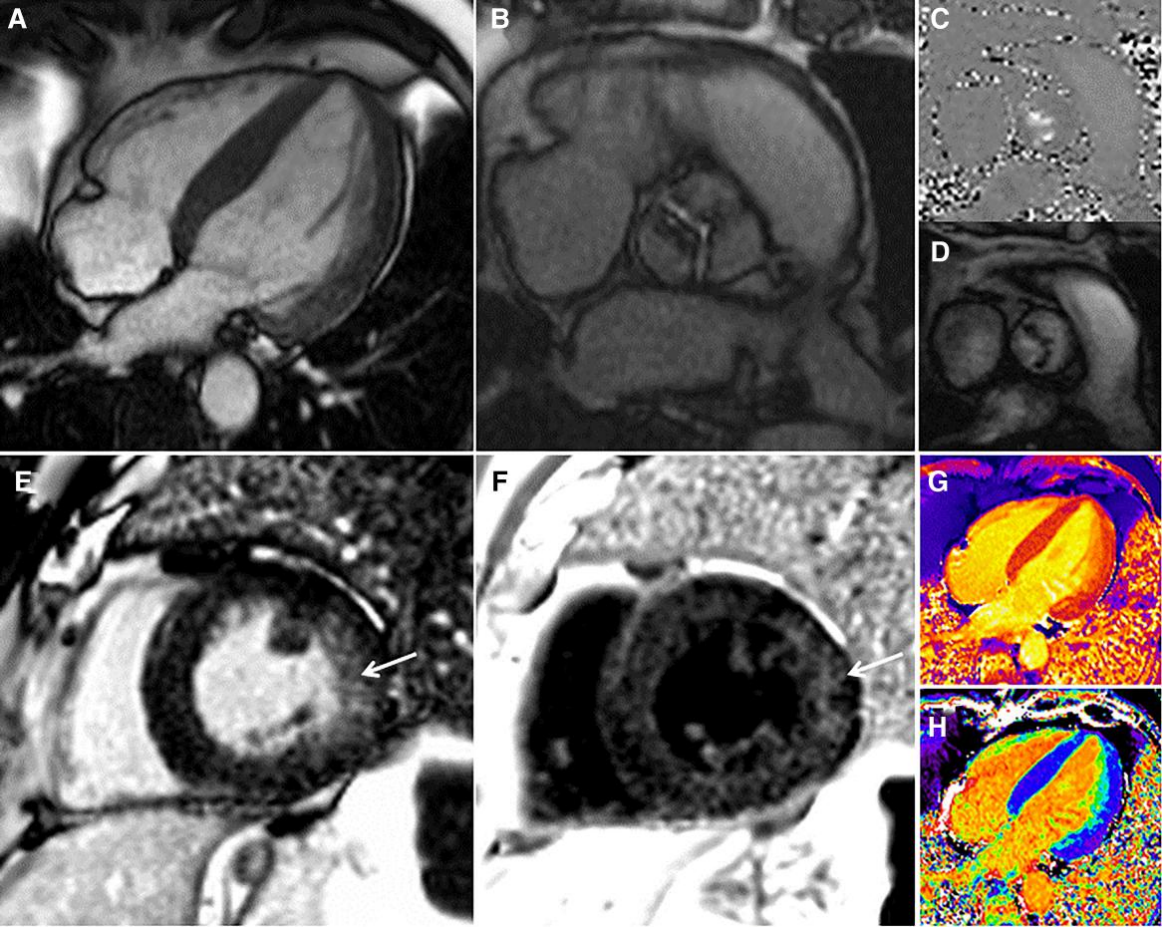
Assessment by magnetic resonance imaging of a patient with critical aortic stenosis. A 4-chamber bSSFP-cine image (*A*) showing normal left ventricular cavity size with concentric hypertrophy. Short-axis bSSFP-cine image (*B*) en-face view of the aortic valve demonstrating fusion of the left and right coronary cusp and a planimetered aortic valve area of 0.6 cm^2^. Phase-contrast imaging just above the aortic valve (*C* + *D*) demonstrating a peak velocity of nearly 5 m/s. Bright (*E*) and dark (*F*) blood late gadolinium enhancement images demonstrating patchy, non-infarct scar in the lateral wall. A native T1 map (*G*) and extracellular volume fraction map (*H*) demonstrate no evidence of myocardial infiltration (septal T1 1040 ms, lateral wall T1 1060 ms, normal <1060 ms; septal ECV 26%, lateral wall ECV 28%, normal <30%).
